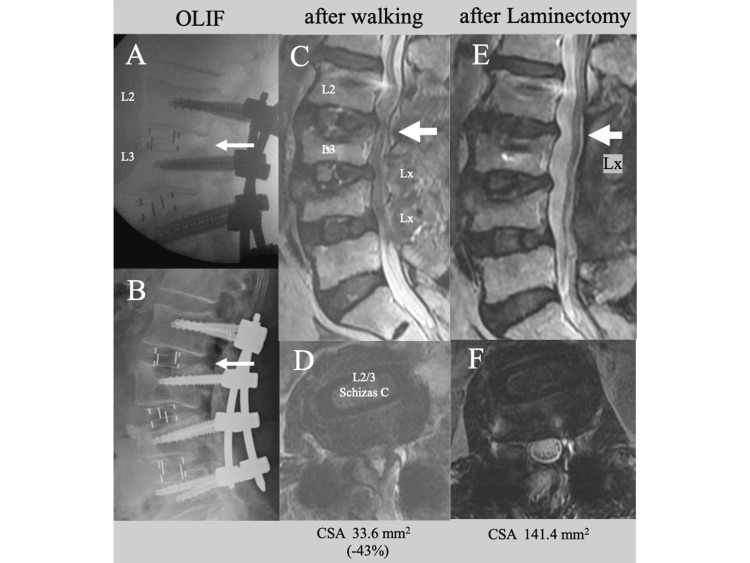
Postoperative lateral lumbar X-rays and MRI images of Case 1 A lateral lumbar X-ray (A) taken immediately after OLIF with pedicle screw fixation showing restoration of PDH at L2/3 (arrow), (B) subsequent advancement in cage subsidence with a decrease in PDH (Marchi grade I, arrow) after commencing walking, and postoperative MRI images showing (C) a narrow dural sac (large arrow) and (D) a 43% decrease in CSA. Following direct posterior decompression at L2/3 (large arrow in E), MRI images (E, F) showing an enlarged dural sac. OLIF: oblique lumbar interbody fusion, PSF: pedicle screw fixation, PDH: posterior disc height, CSA: cross-sectional spinal canal area
